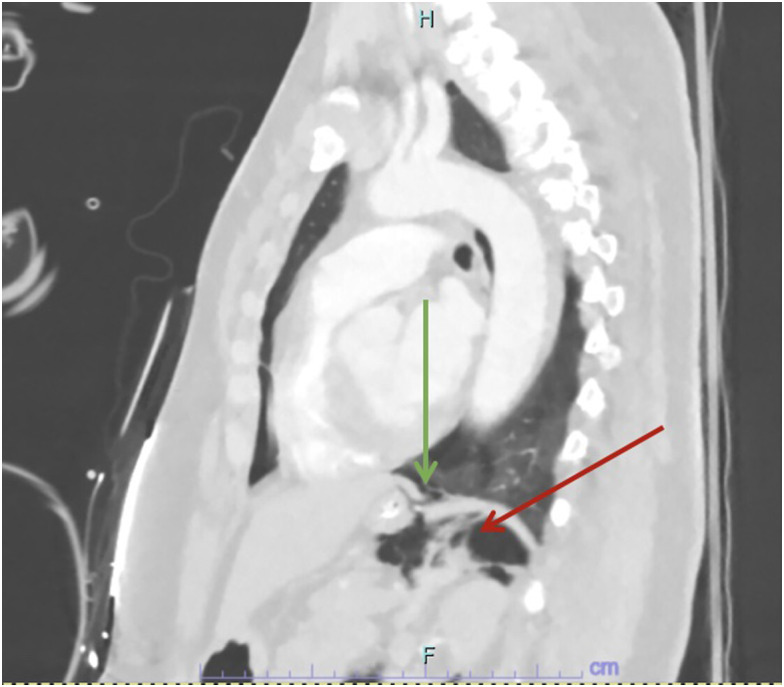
The initial computed tomography with pulmonary embolism protocol performed on the day of presentation demonstrated discontinuity of the medial left hemidiaphragm posteriorly (green arrow) with air tracking from the lower mediastinum into the left retroperitoneal space posterior to the stomach (red arrow).
